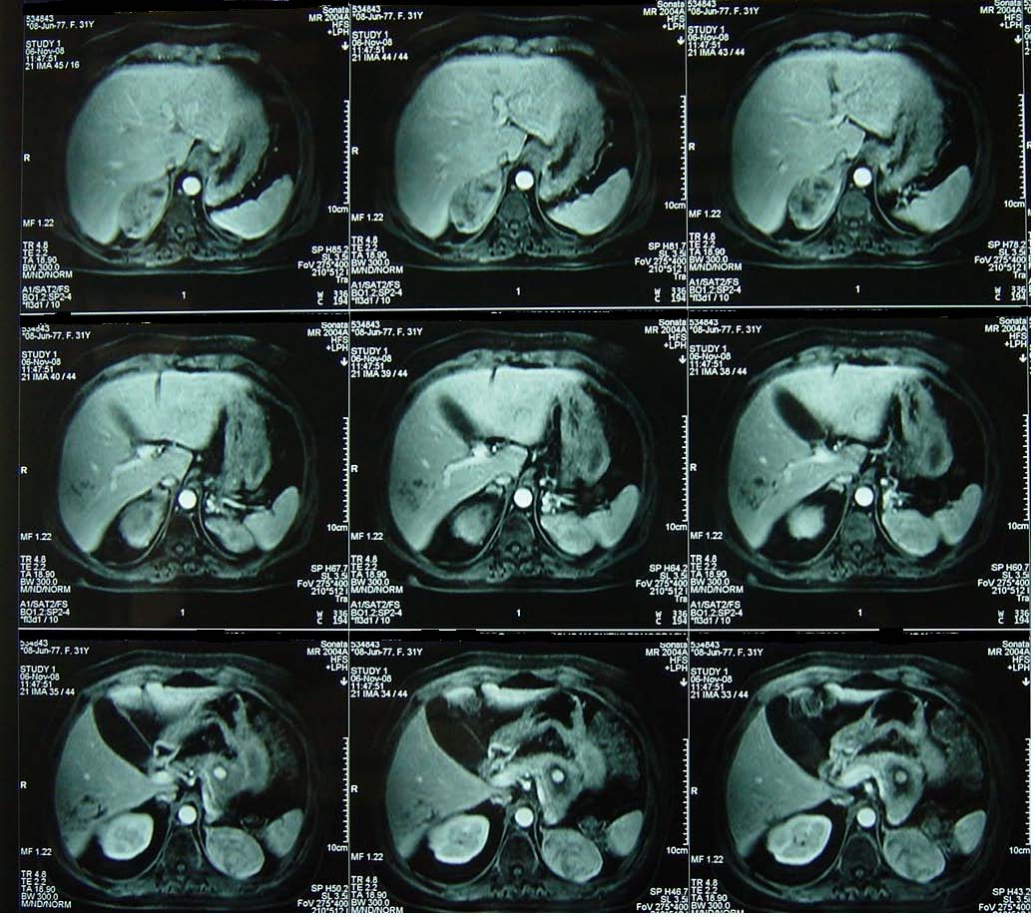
MRI scan of the upper abdomen disclosing bilateral adrenal tumors.
